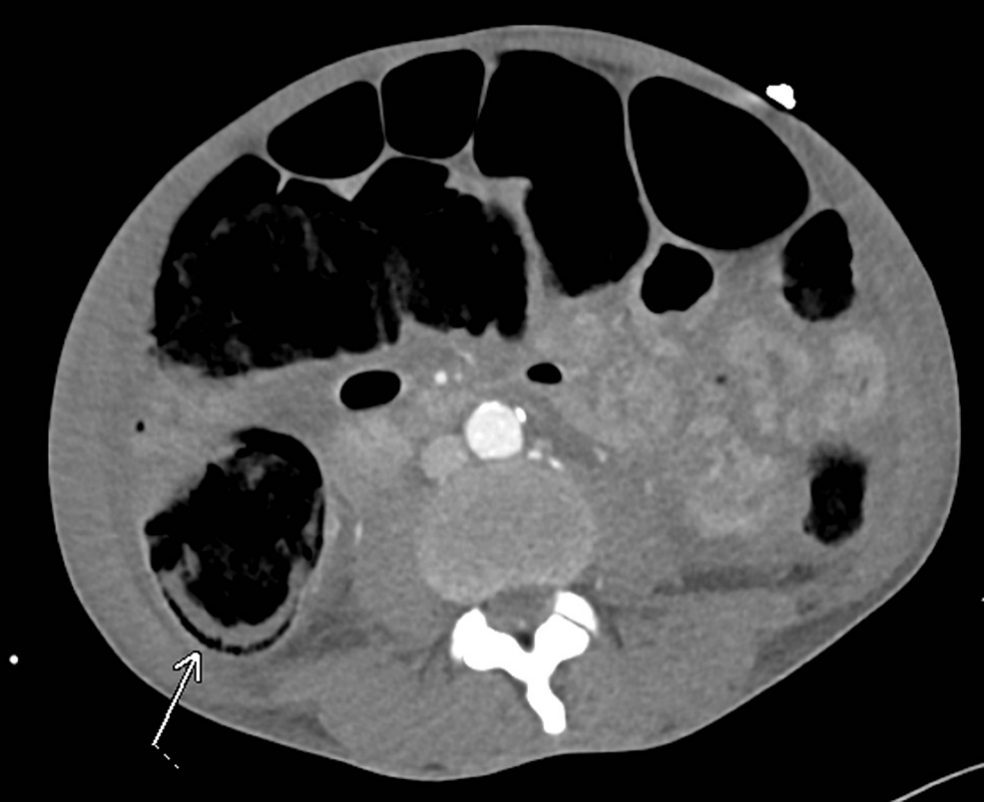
CT abdomen with pneumatosis
